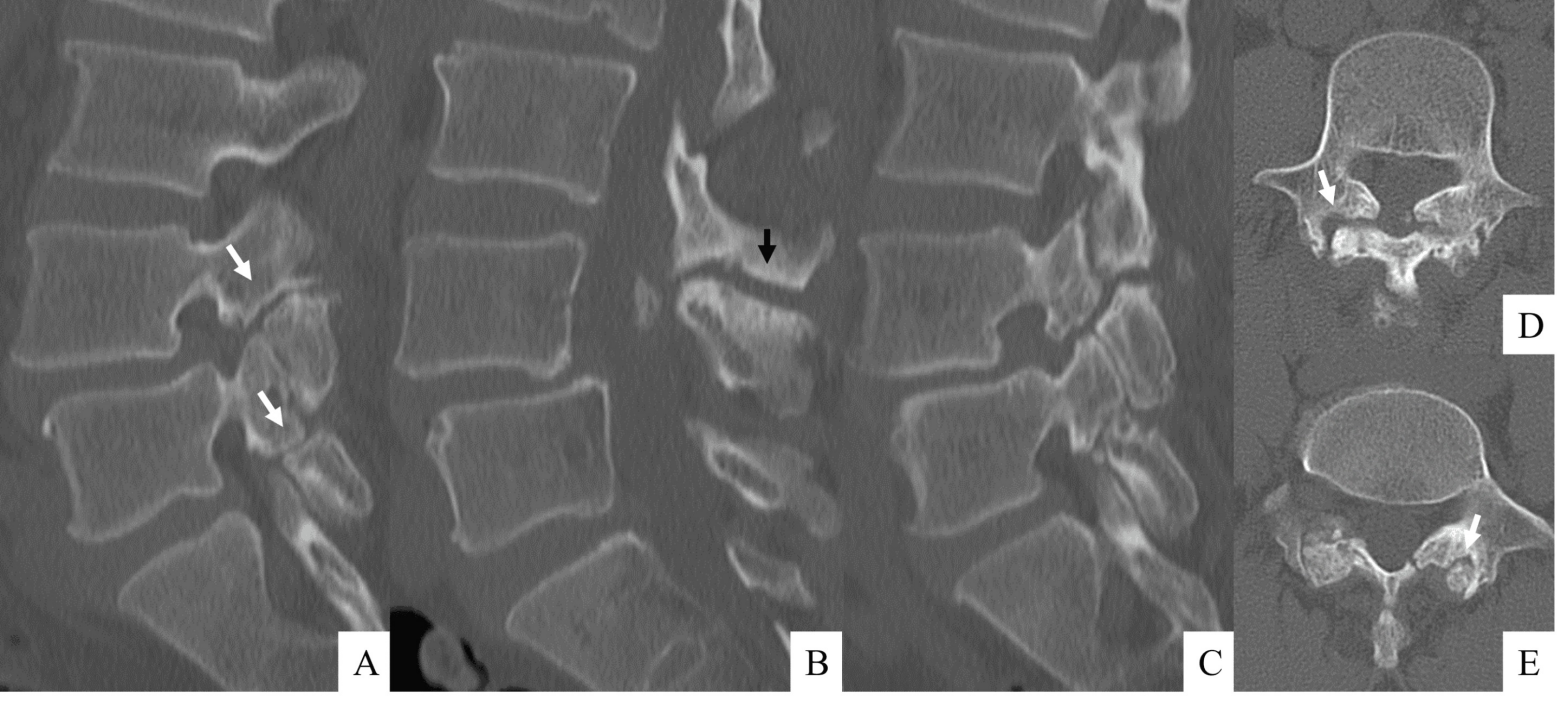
Preoperative computed tomography images A computed tomography scan reveals the presence of spondylolysis both at L4 with spondylolisthesis and at L5 with minimal slippage (A-C; from the right parasagittal to the left parasagittal view, D, E; L4 and L5 axial view, respectively. The white arrows indicate spondylosis.). Additionally, the scan demonstrated evidence of bony sclerosis and erosion between the L3 and L4 spinous processes (B; black arrow).
